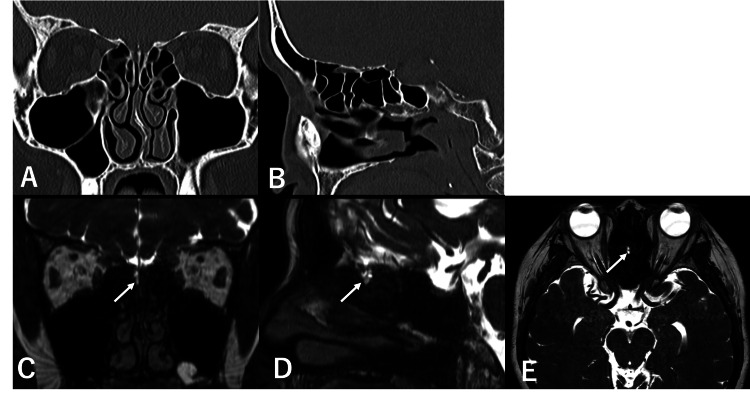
Preoperative CT and MRI findings (case 2) On preoperative (A) coronal and (B) sagittal CT scans, an area on the right cribriform plate appeared to be a bone defect, but this could not be definitively confirmed. (C) Coronal, (D) sagittal, (E) axial MRI CISS showed a high signal on the right cribriform plate (white arrow). CT, computed tomography; MRI, magnetic resonance imaging; CISS, constructive interference in steady state
